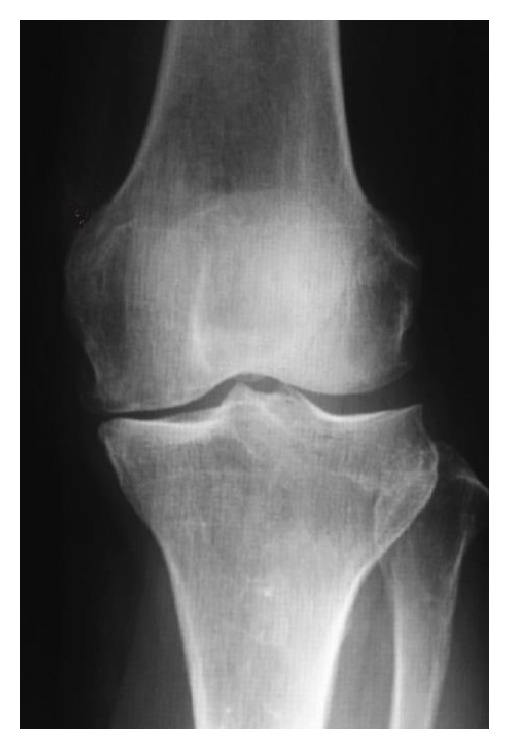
Preoperative plain radiograph of Case 2 shows slight degenerative changes, suggesting Kellgren-Lawrence grade 2.
